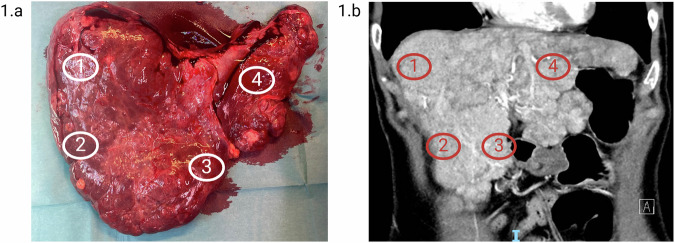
Images of liver. a Extracted liver displaying visible arteriovenous malformations (AVMs) and multiple focal nodular hyperplasias (FNH). b Coronal contrast-enhanced CT scan in the arterial phase, showing the liver before transplantation with clearly visible intrahepatic arteries and multiple FNH lesions. Biopsies were taken from marked areas: (1) HAVM in hepatic segment 7 (sample ID: G142-3 and G142-4), (2) HAVM in hepatic segment 6 (sample ID: G142-1 and G142-2), (3) HAVM in hepatic segment 5 (sample ID: G142-5 and G142-6), and (4) macroscopic normal tissue in hepatic segment 4 (sample ID: G142-N1 and G142-N2). Created with BioRender.com.
